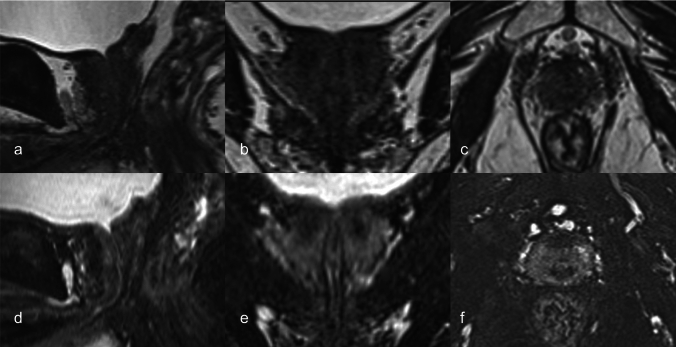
Images of a 68-year-old male in Case 7. The DSC value of the conventional 3D T2-weighted image was 0.70, and the visibility score was 2 (a–c). The urethra is a little difficult to visually identify. In contrast, the DSC value of the proposed fat-suppressed 3D T2-weighted image was 0.79, and the visibility score was 4 (d–f). The curve-reconstructed coronal image (e) shows a quite distinct boundary urethra/prostate
